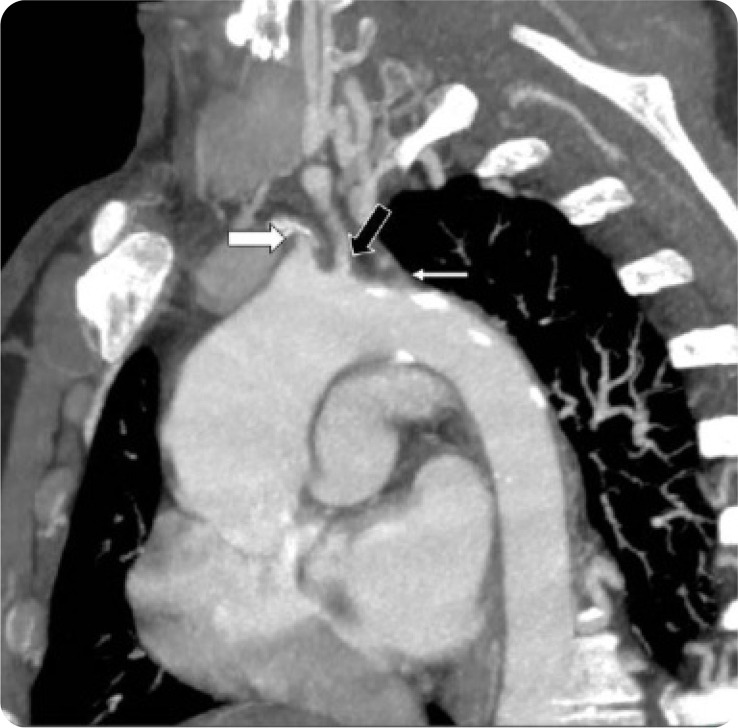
CT Chest MIP “candy cane” reformat demonstrating occlusion of the right brachiocephalic (thick white arrow) and left subclavian (thin white arrow) arteries and severe stenosis of the left common carotid artery (thick black arrow).
